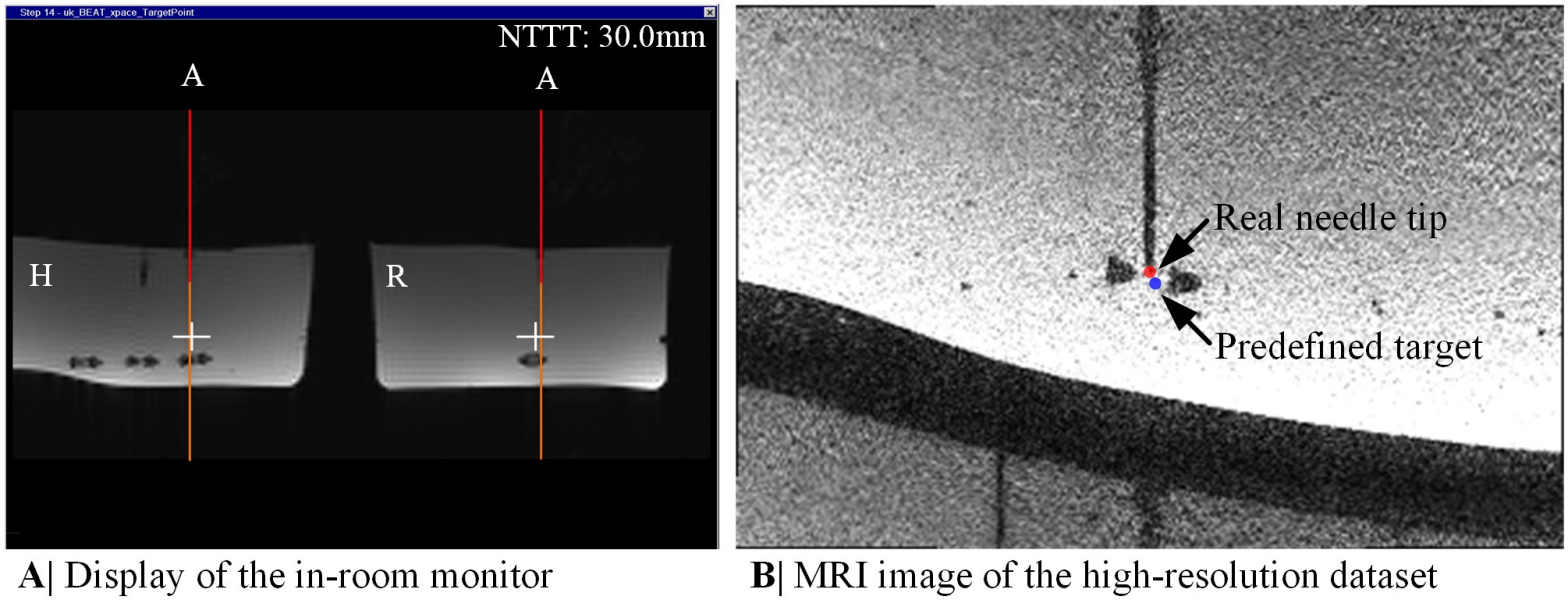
Real-time needle guidance with the MPT system during the user study. (A) The display of the in-room monitor presents images of the real-time needle guidance sequence during the user study together with its graphical overlay. (B) The present MRI image was extracted from one high-resolution dataset. The colored dots mark the detected real needle tip position (red dot) and the predefined target (blue dot).
